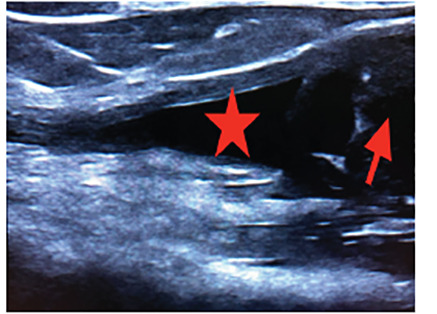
Point-of-care ultrasound in the longitudinal view. The star indicates fluid collection, where the proximal long head biceps tendon (LHBT) is normally seen. The arrow indicates the retracted portion of the LHBT indicating rupture.
